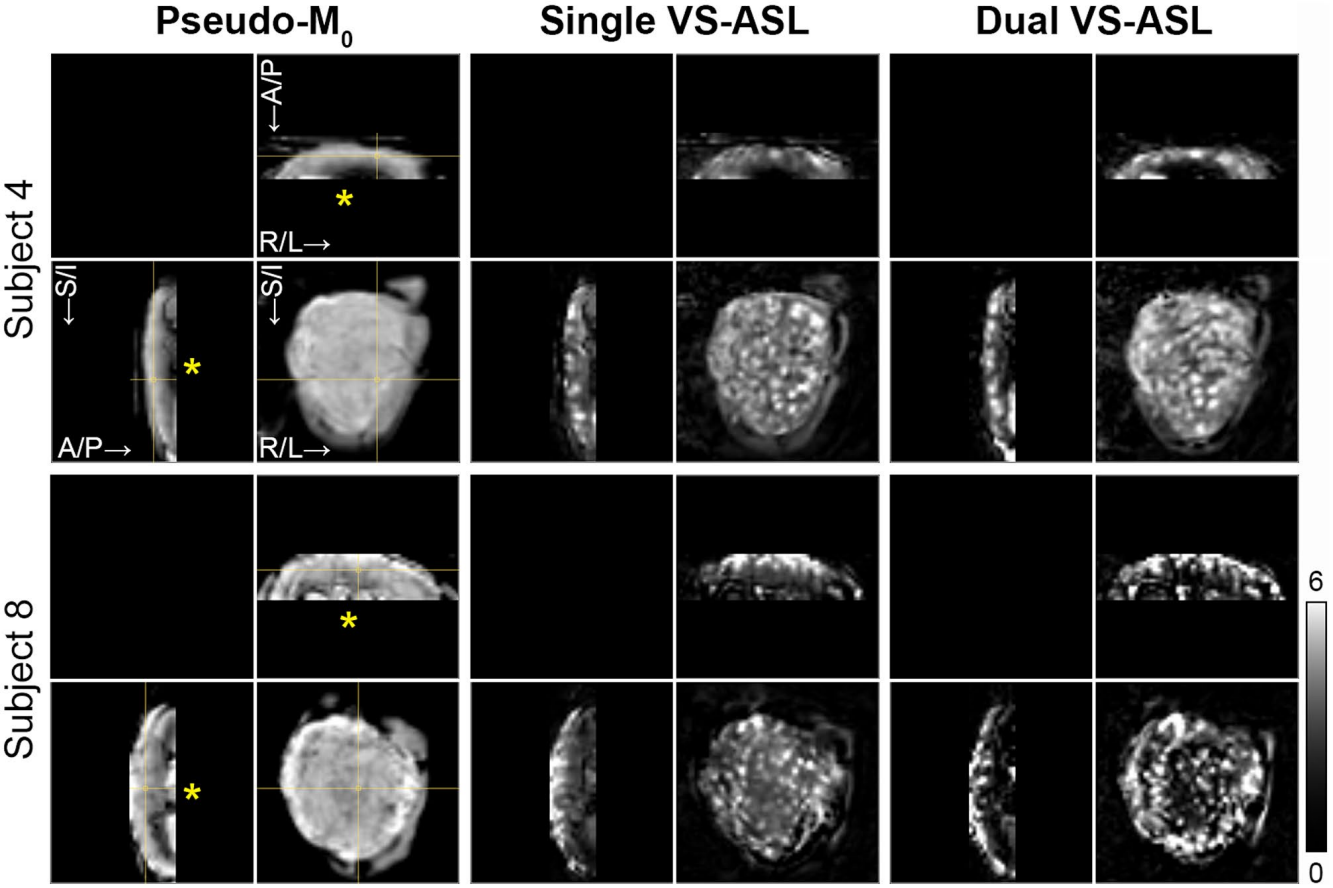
Comparison of single vs. dual VS‐ASL. Placental perfusion images obtained with single (middle column) and dual (right column) VS‐ASL MRI and the corresponding pseudo‐M_0_ images (left column) in two pregnant volunteers (Subject 4 and 8 in Table 1). Orthogonal slices (spatial resolution: 4 × 4 × 4 mm^3^) are shown. The cross‐hair in the pseudo‐M_0_ images indicates the intersection of the slices. The yellow asterisk on the axial and sagittal slices point at the fetal chorionic side of the placenta. PWS images have equal LUT scaling within each subject. The grayscale bar indicates PWS [%]. A/P, anterior‐posterior; R/L, right‐left; S/I, superior‐inferior
